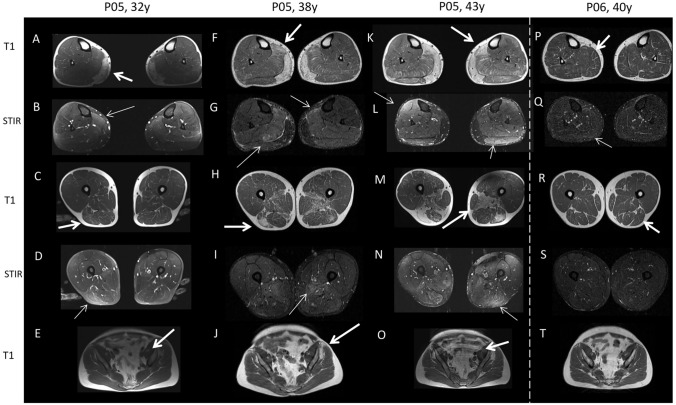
Longitudinal MRI data of the cohort. A Progression of the total lower-body (TLB) score between the first and last MRI. The dashed line represents the change from a pseudometabolic to LGMD-R12/MMD3 phenotype; dotted lines represent the change from asymptomatic to LGMD-R12/MMD3; the double line represents scores reduced by half for visibility purposes. B Number of muscles with and without STIR hyperintensities and their progression of fat infiltration across subsequent MRIs. C Progression of TLB and compartment-specific T1 scores. The left bar represents the first MRI, and the right bar the last available MRI. Colour coding: lower leg (dark grey), thigh (medium grey), pelvis (light grey). The total bar height represents the TLB score. Values for patient P01 were reduced by half for visibility purposes; *y* years, *M* male, *F* female
